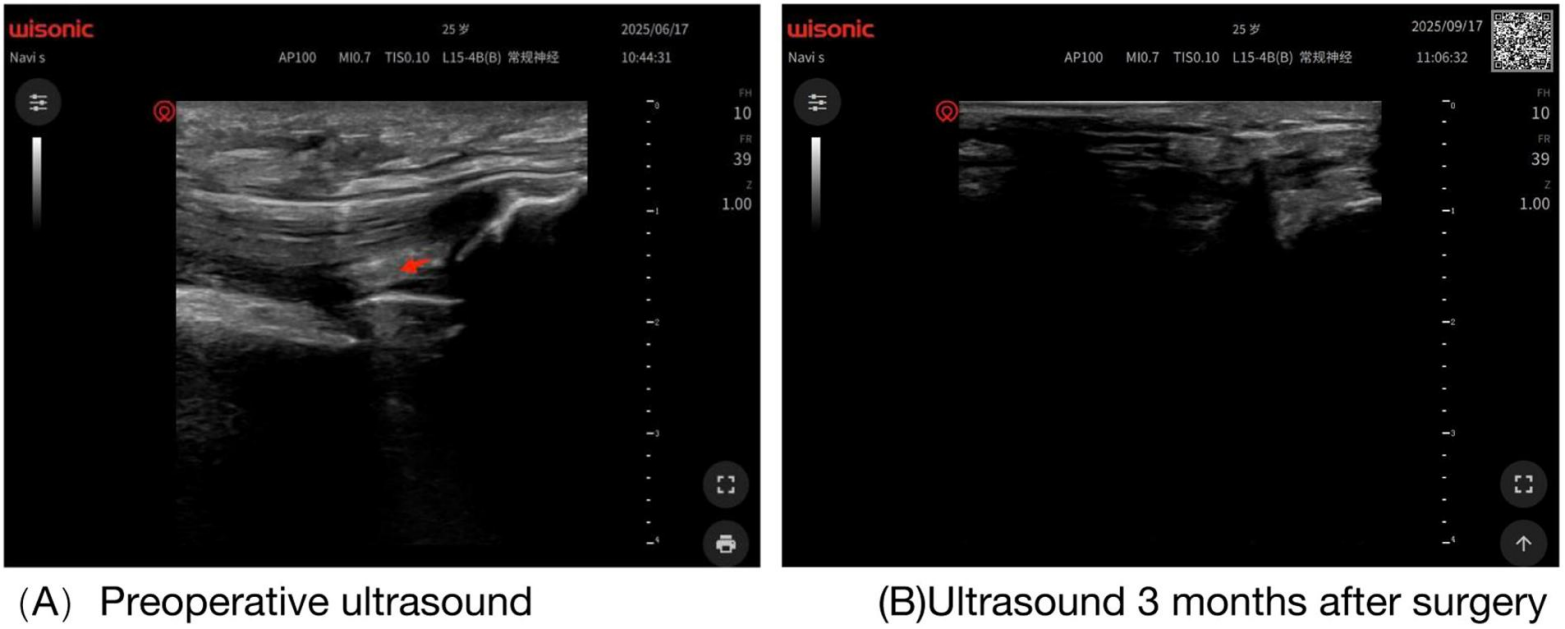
(A) Preoperative knee ultrasound image (red arrow indicates adhesion site). (B) Knee ultrasound image three months postoperatively.
